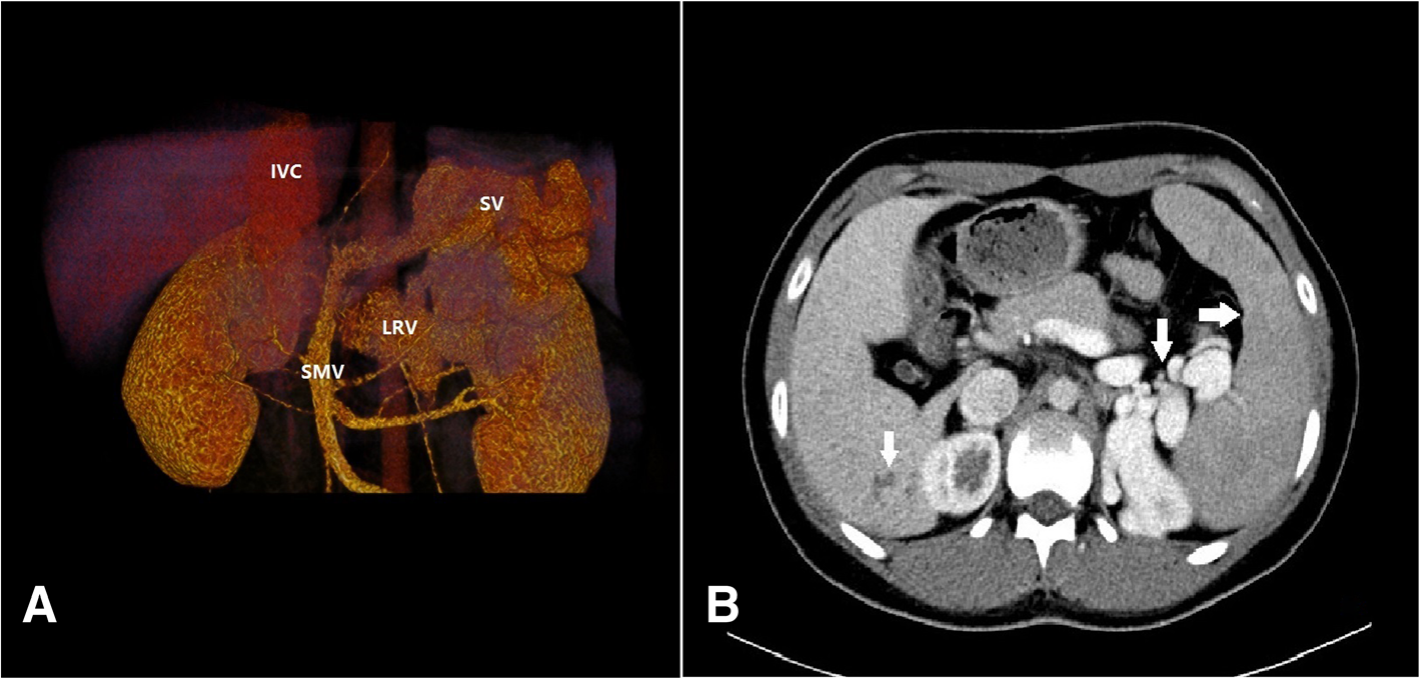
PV-CTA shows that the trunk and branches of PV are absent, the SV is widened, with the SMV joining the LRV and then draining directly into the IVC without passing through the liver (a). CTA also reveals the enlargement of the spleen and splenic veins (arrows) and heterogeneous density lesions in the liver nodules (arrows) (b). PV: portal vein; CTA: computed tomography angiography; SV: splenic vein; SMV: superior mesenteric vein; LRV: left renal vein; IVC: inferior vena cava
